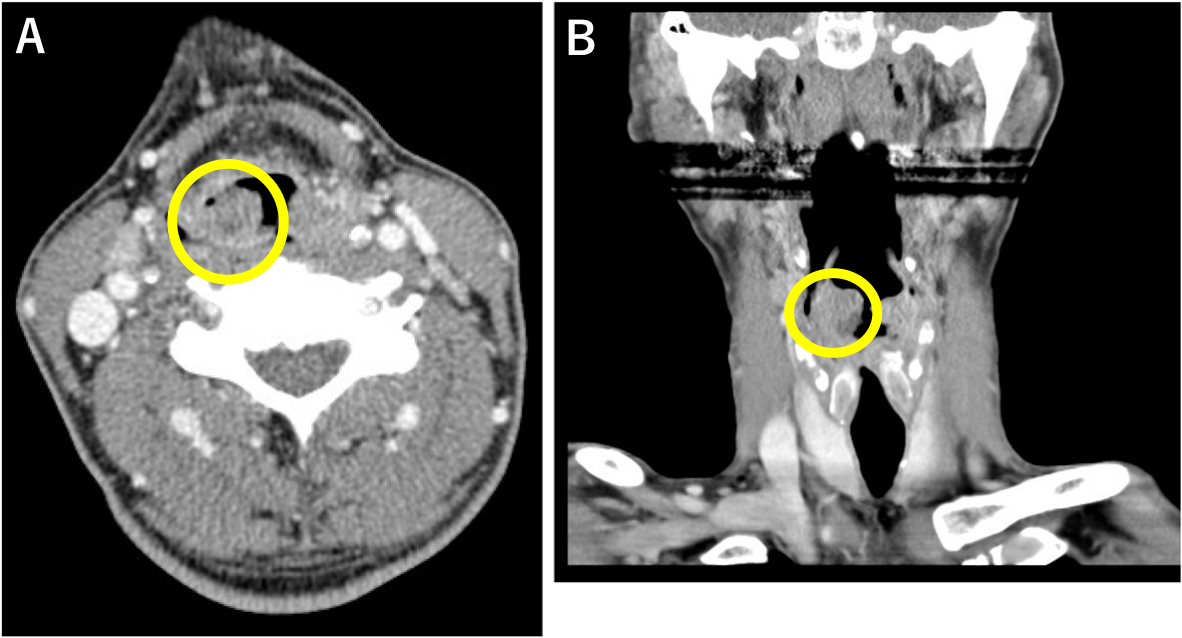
a: Axial section of head and neck computed tomography. b: Coronal section of head and neck computed tomography. Both images show the right arytenoid edema (yellow circle)
